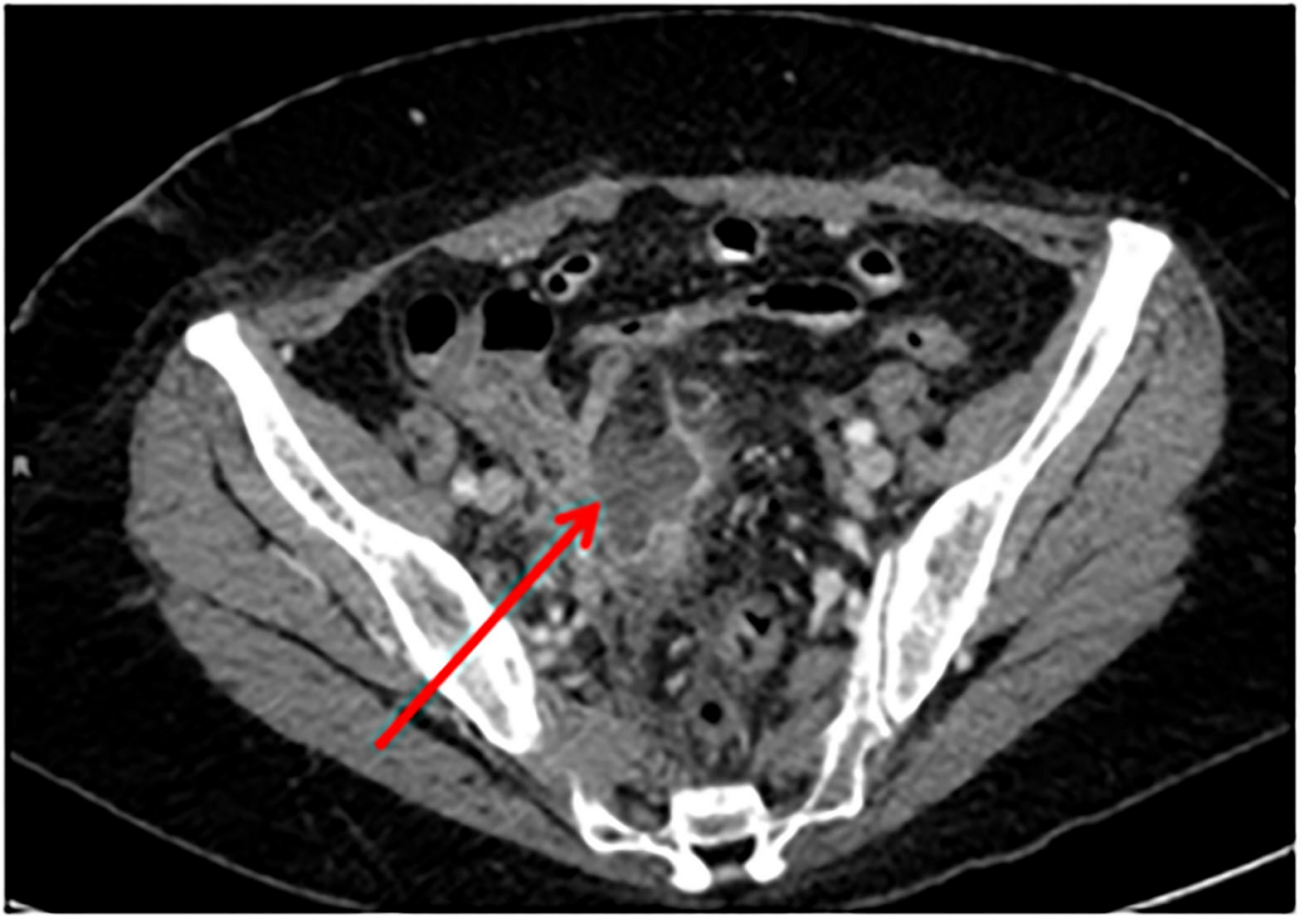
CT: purulent collection of the pelvis between the vagina and the rectum (arrow). CT, computed tomography.
